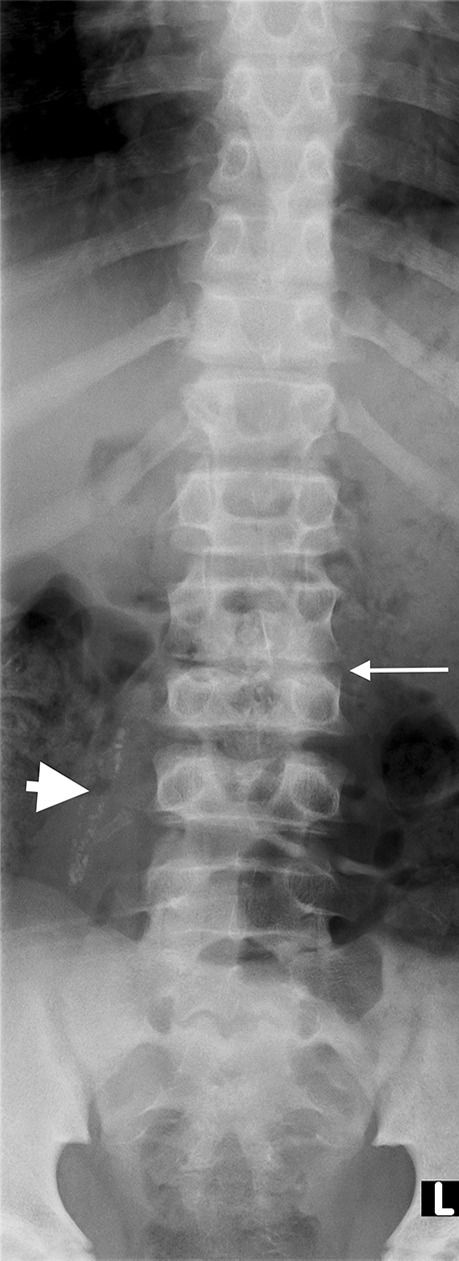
Anteroposterior radiograph of the lumbar spine in a 10-year-old boy with a history of treated tuberculous spondylitis shows disc space narrowing at L2/L3 and loss of height of L3 with sclerosis of its superior endplate (*thin arrow*). There is calcification of the right psoas muscle (*thick arrow*)
